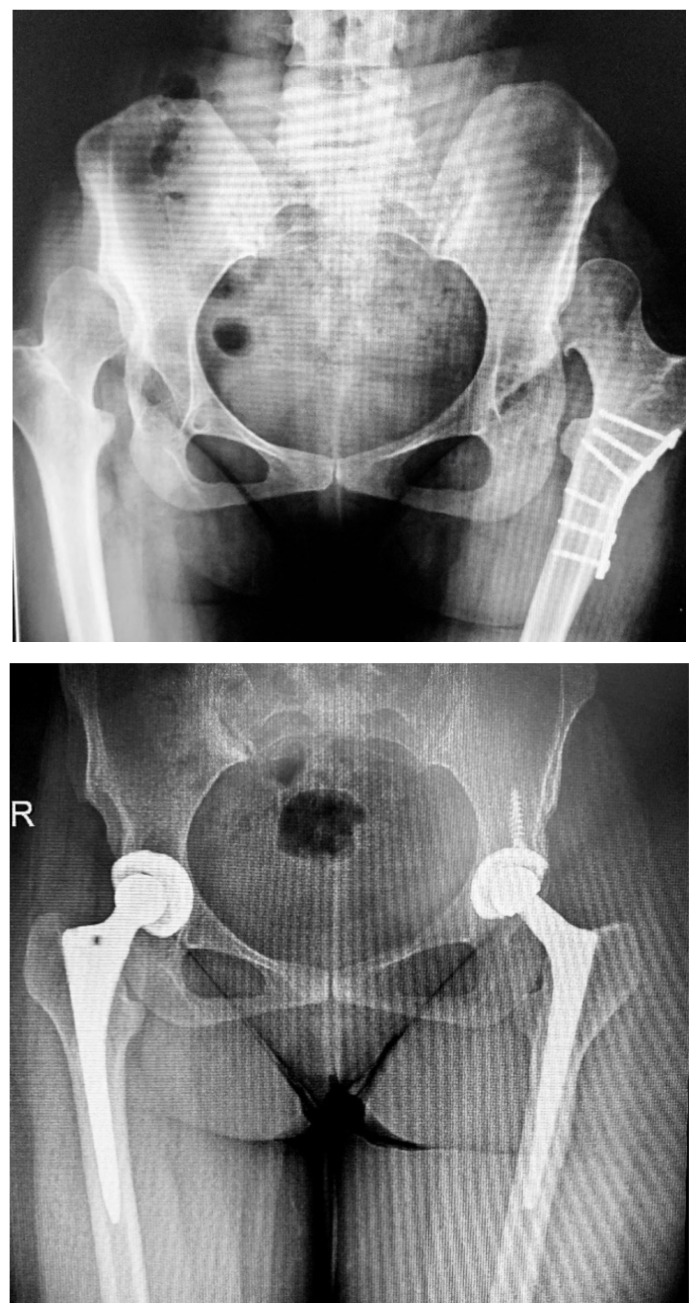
(a) Preoperative X-ray of bilateral Crowe type III hips. (b) Postoperative X-ray of bilateral THA taken 6.8 years later. The right hip was treated with the press-fit technique, while the left hip was fixed with screws.
